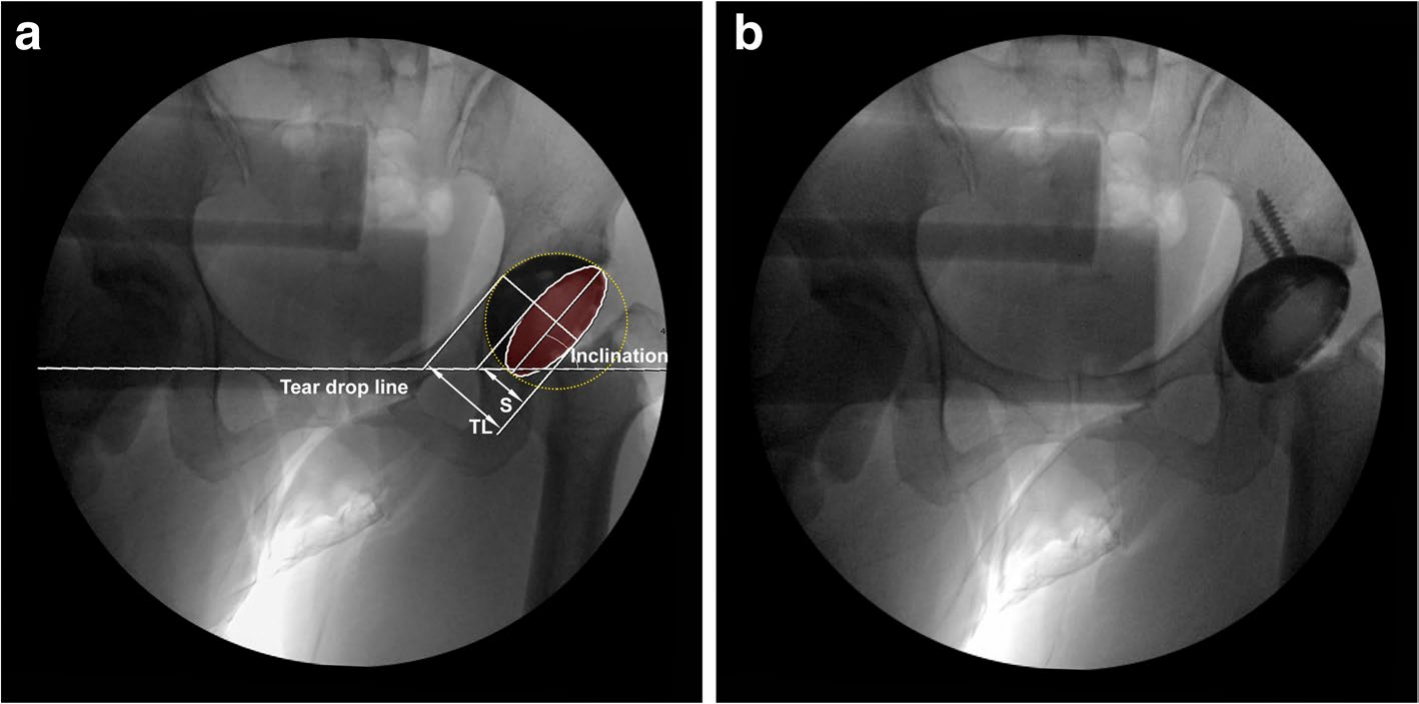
A single anteroposterior (AP) hip X-ray was taken intraoperatively after temporary insertion of acetabular component (a). Another anteroposterior (AP) hip X-ray was taken if adjustments were made, the next step, screws insertion and fixation of the acetabular component was proceeded (b)
